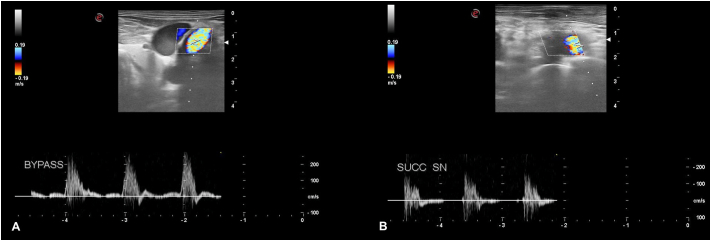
Ultrasound doppler showing patency of Bypass (A) and left carotid-subclavian bypass (B).
